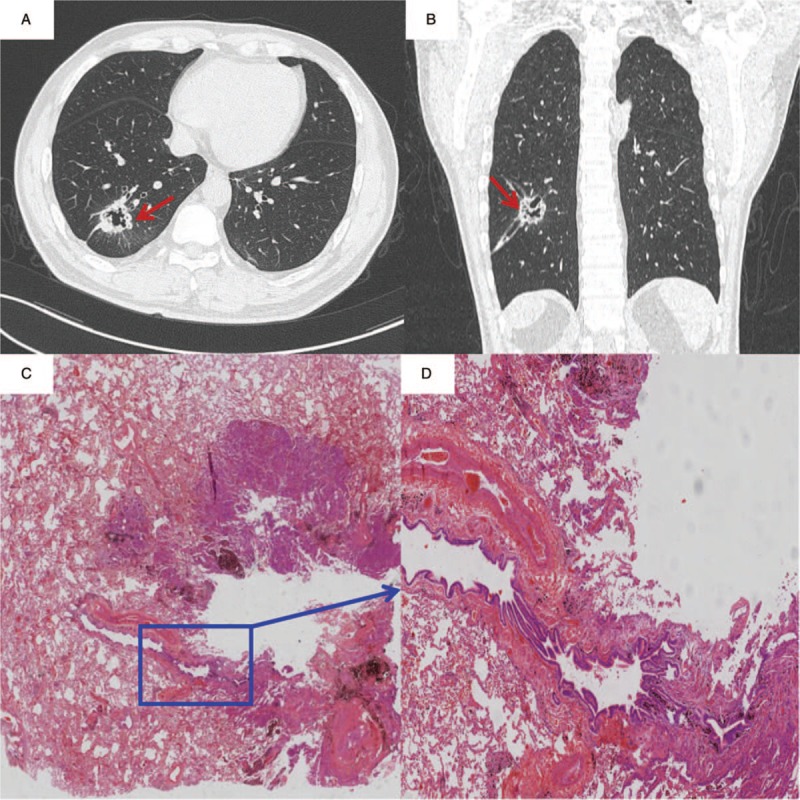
On the computed tomography film of a 46-year-old male patient, we can see tracheal and vascular structure on the wall of the cavity (A and B). On the pathologic scanning, we can clearly see the boundary of the tumor (C) and see that the bronchus is connected to the cavity (D).
